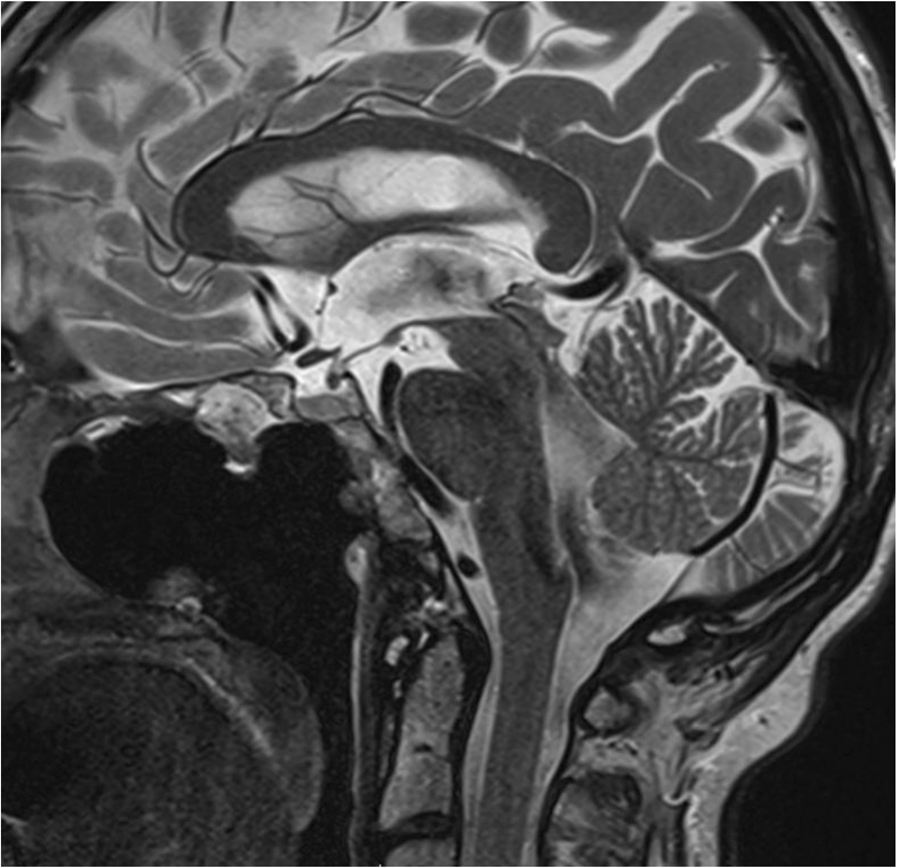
T2-weighted sagittal magnetic resonance scan shows total excision of the lesion with the packing material seen in the posterior part of the clivus in the extradural plane.
